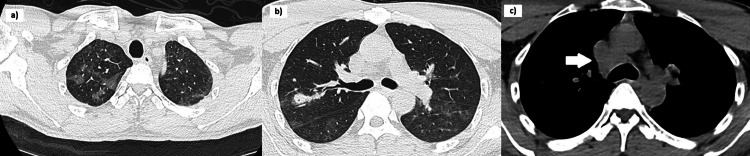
A CT scan of the thorax, abdomen, and pelvis shows ground glass opacities mainly distributed in a) both upper lobes, b) the superior segment of the lower lobes, and c) enlarged right lower paratracheal lymph nodes of 1.6 cm.
